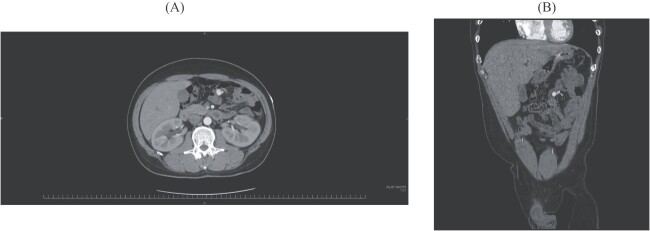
(A) Axial image of CE-CT shows a round, hypervascular nodule. (B) Coronal image shows vascular dilatation in the mesentery close to the nodule.
